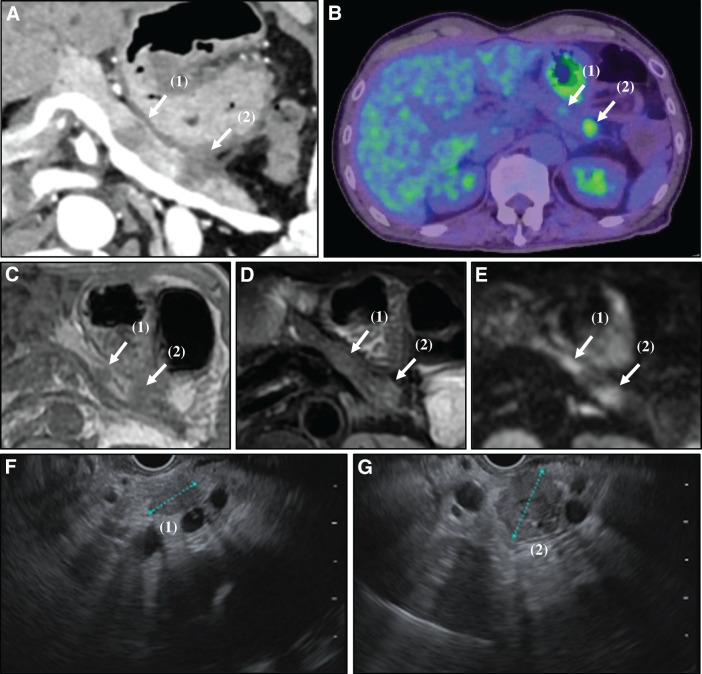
Imaging of remnant pancreatic lesions 7 years after initial cholangiocarcinoma resection. (A) Contrast-enhanced abdominal CT (arterial phase) demonstrating 2 hypovascular lesions in the remnant pancreatic tail. (B) FDG-PET-CT showing 2 areas of FDG uptake in the pancreatic tail tumors. MRI demonstrating 2 lesions in the remnant pancreas with low signal intensity on T1-weighted imaging (C), high signal intensity on T2-weighted imaging (D), and high signal intensity on diffusion-weighted imaging (E). (F, G) EUS demonstrating 2 hypoechoic masses in the remnant pancreas. White arrows indicate lesions (1) and (2), and blue dotted lines in panels (F) and (G) indicate their measured diameters. EUS, endoscopic US
